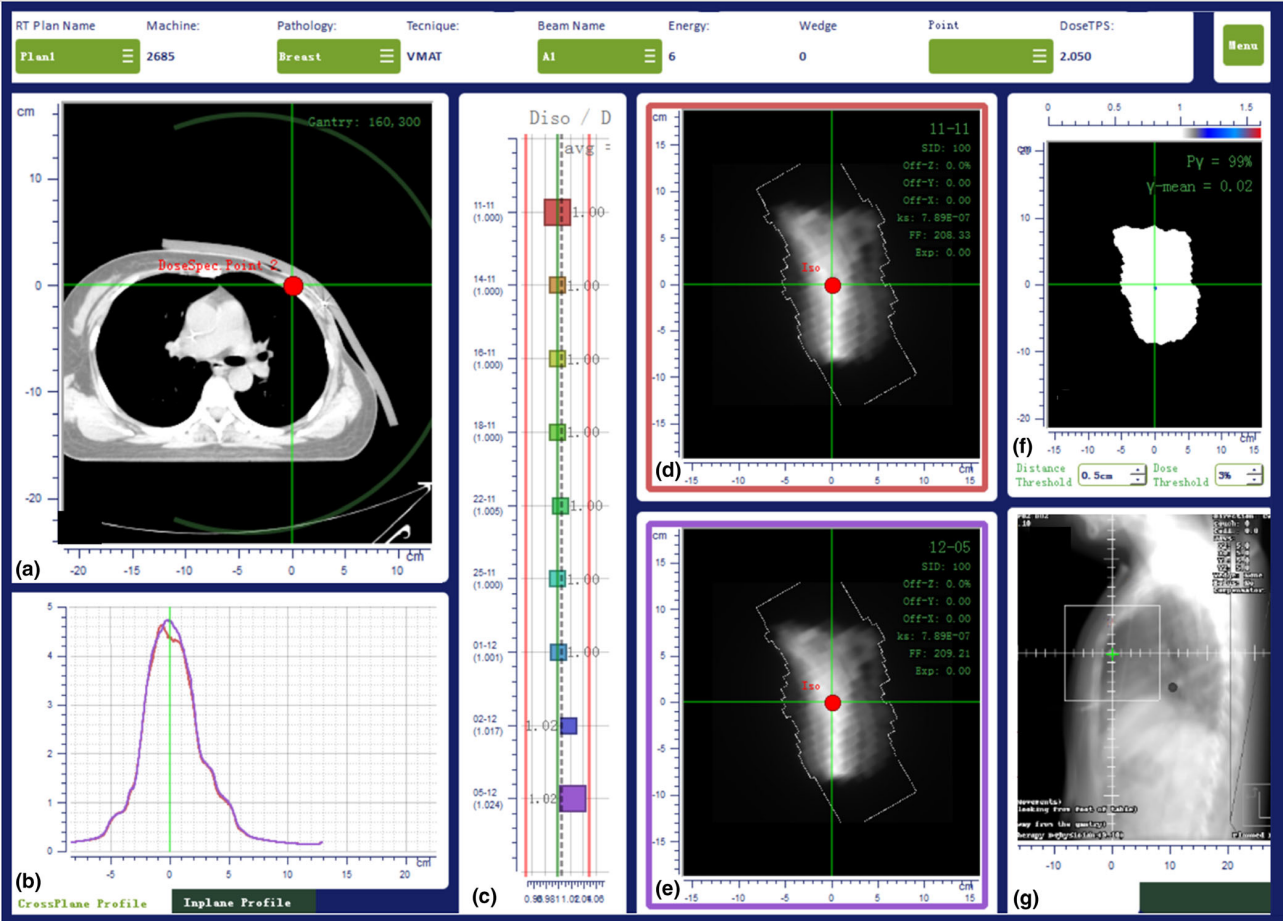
The result of interfractional variation using the EPID‐based IVD. (a) patient CT and planned isocenter, (b) signal profiles and (c) ratio between planned and daily image, (d) reference and (e) daily IVD image, (f) map of points with γ > 1, and (g) map of points with γ > 1 on the sagittal digital reconstructed radiography.
